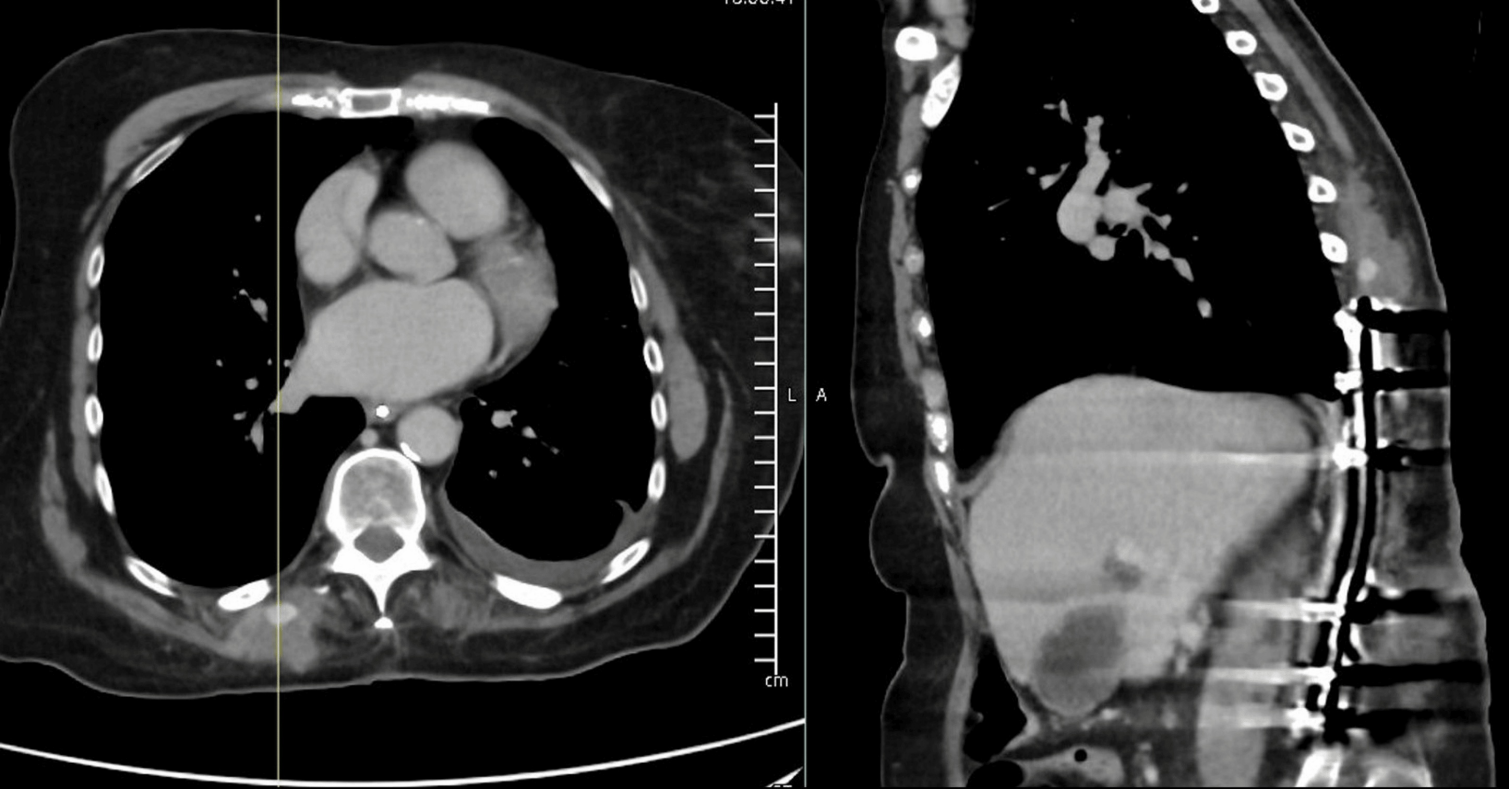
CT angiography demonstrating pseudoaneurysm Axial (left) and sagittal (right) CT angiography images showing a well‑defined intramuscular hematoma adjacent to the pedicle screw tract. The axial image includes a reference line indicating the corresponding sagittal level. Imaging features are consistent with a pseudoaneurysm.
